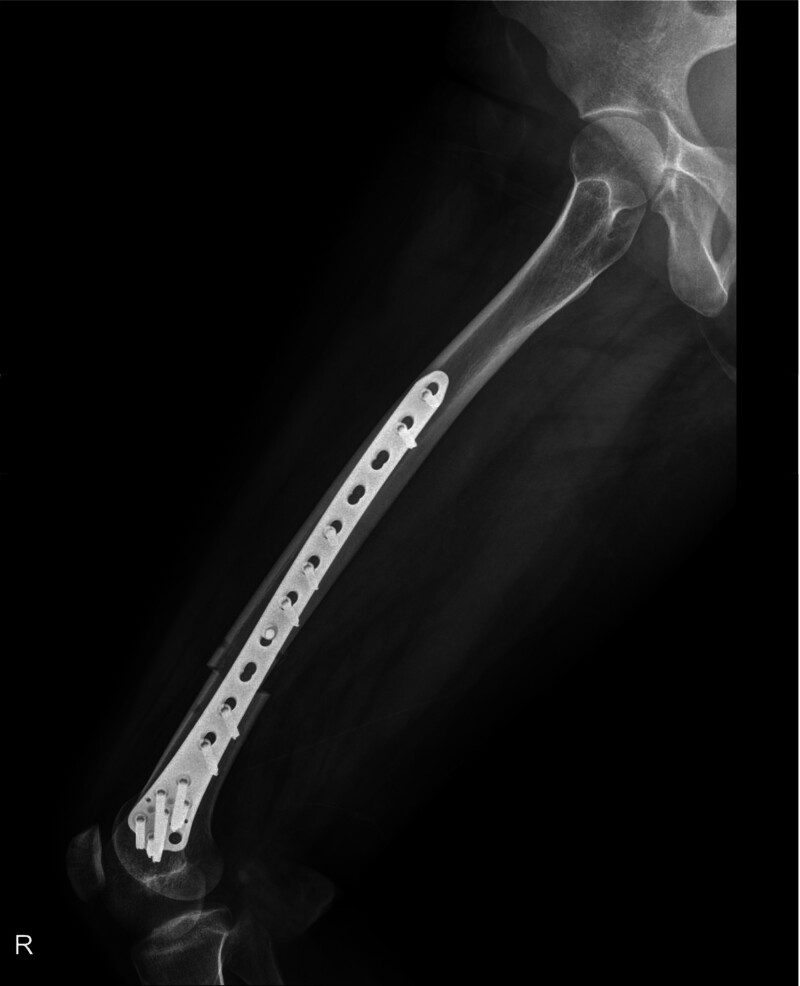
Postoperative radiograph. The osteotomy level was slightly proximal due to the contour of the distal femur and the length of the fixation plate. The small anterior cortical holes correspond to the Schanz pin tracks used intraoperatively to confirm 15° derotation. The cutting plane was oriented from anterodistal to posteroproximal, resulting in unintended femoral valgization.
